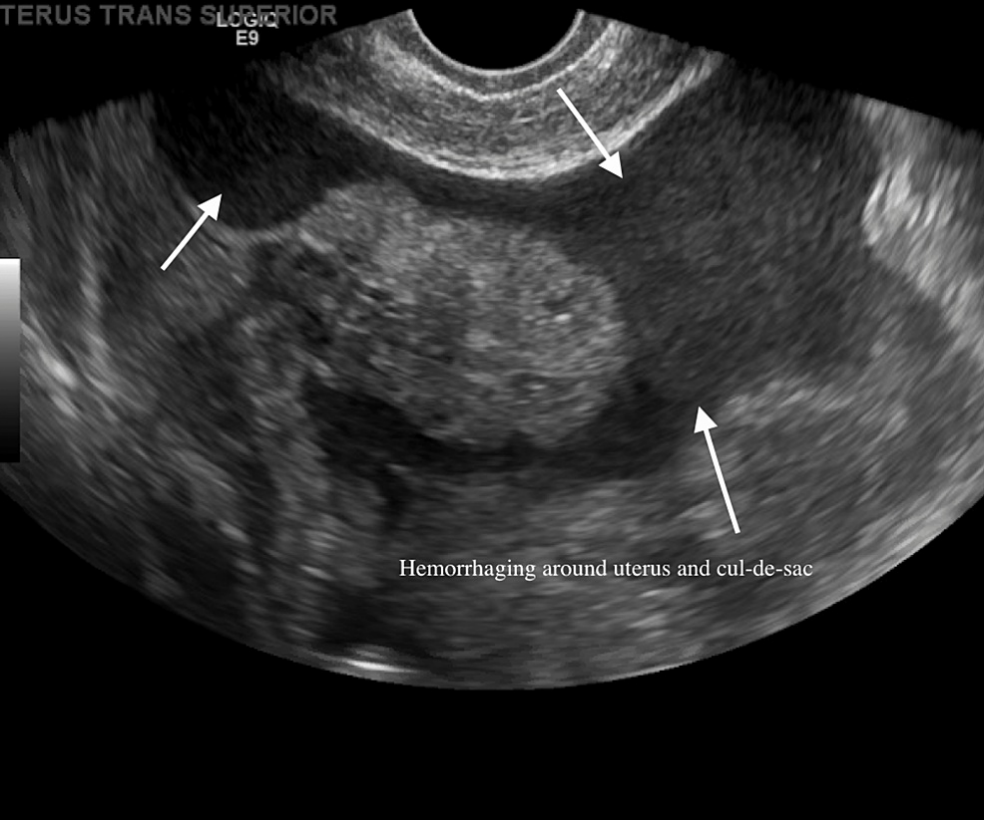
Alternate ultrasound view of hemorrhaging around the uterus and cul-de-sac.
